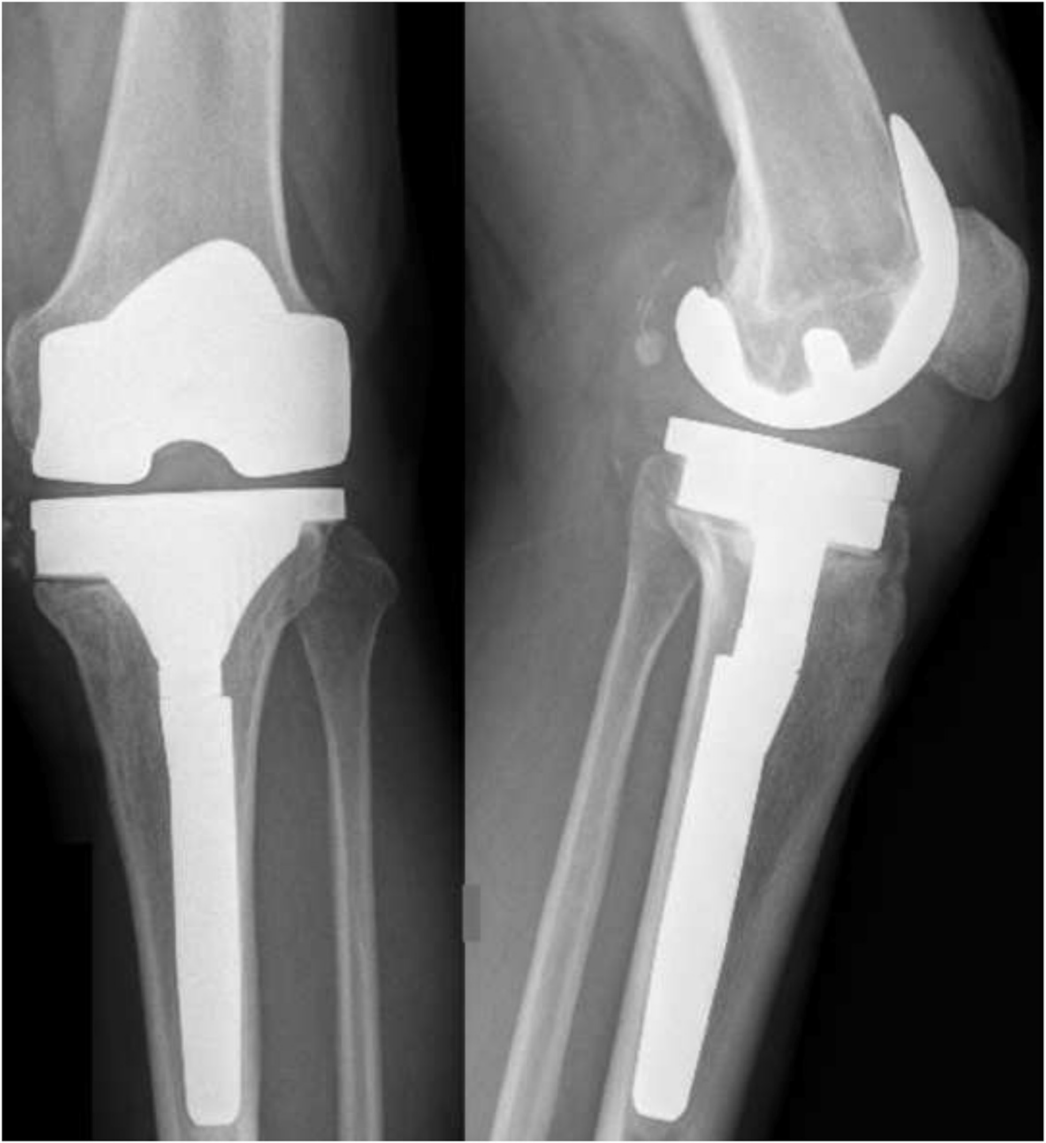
X-rays (AP and lateral view) of knee joint after second stage of revision arthroplasty (revision implants)
